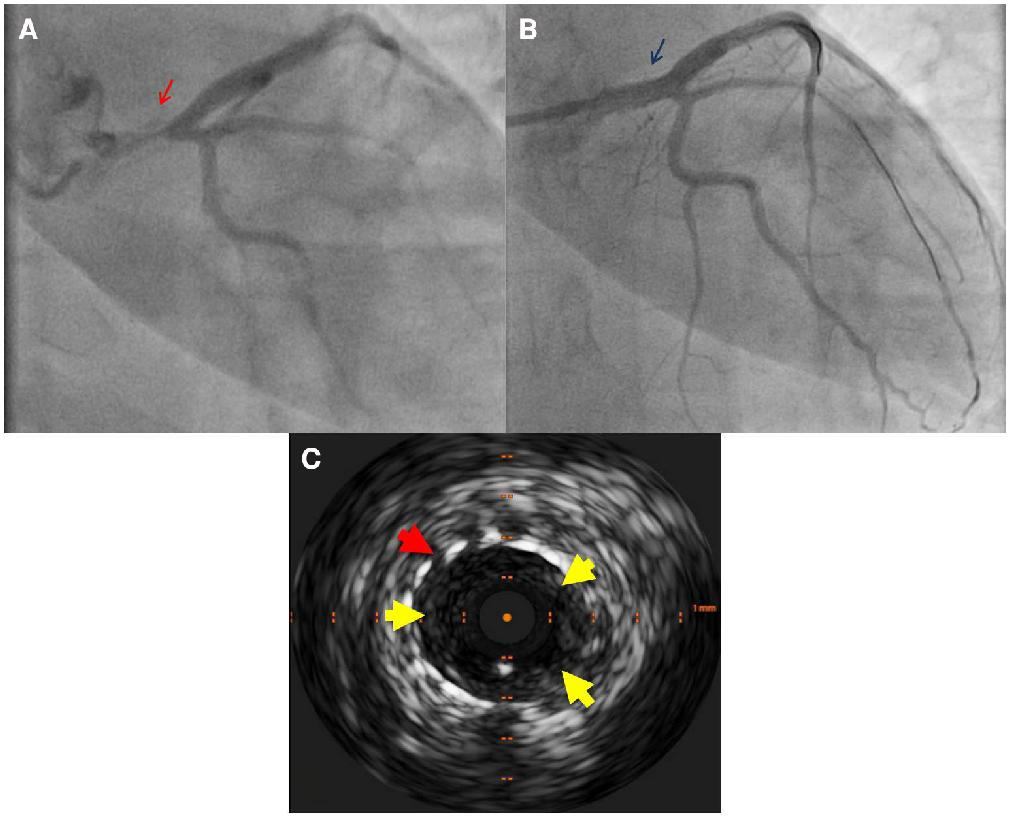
(*A*) Second coronary angiogram demonstrating severe in-stent restenosis of the first left main stem drug-eluting stent, involving the left anterior descending artery ostium (arrow). (*B*) Angiographic result after the second percutaneous coronary intervention (arrow). (*C*) Intravascular ultrasound demonstrating well-apposed stent in the left main stem (outer arrow) with significant neointimal formation (inner arrows); 187 × 232 mm.
